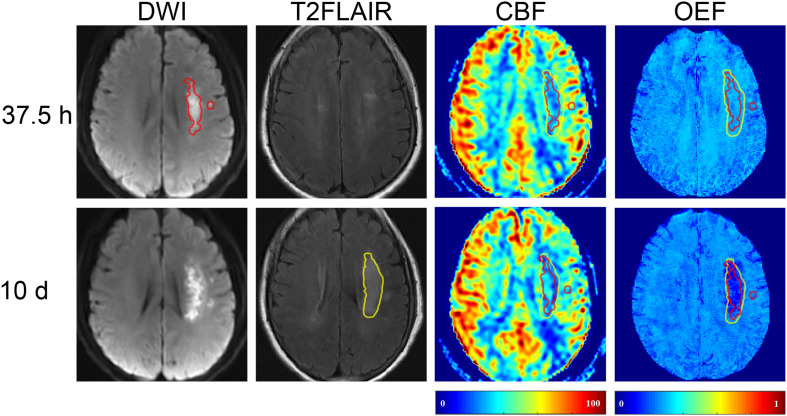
Representative images of a 64-year-old female with right limb weakness and aphasia. The FI (with a volume of 5.31 ml) on 10-day T2FLAIR (shown in yellow) was larger than the 37.5-h IDL (10.97 ml) on DWI (shown in red). The area of FI that did not overlap with IDL was the mismatch. T2FLAIR, T2 fluid-attenuated inversion recovery; DWI, diffusion-weighted imaging.
